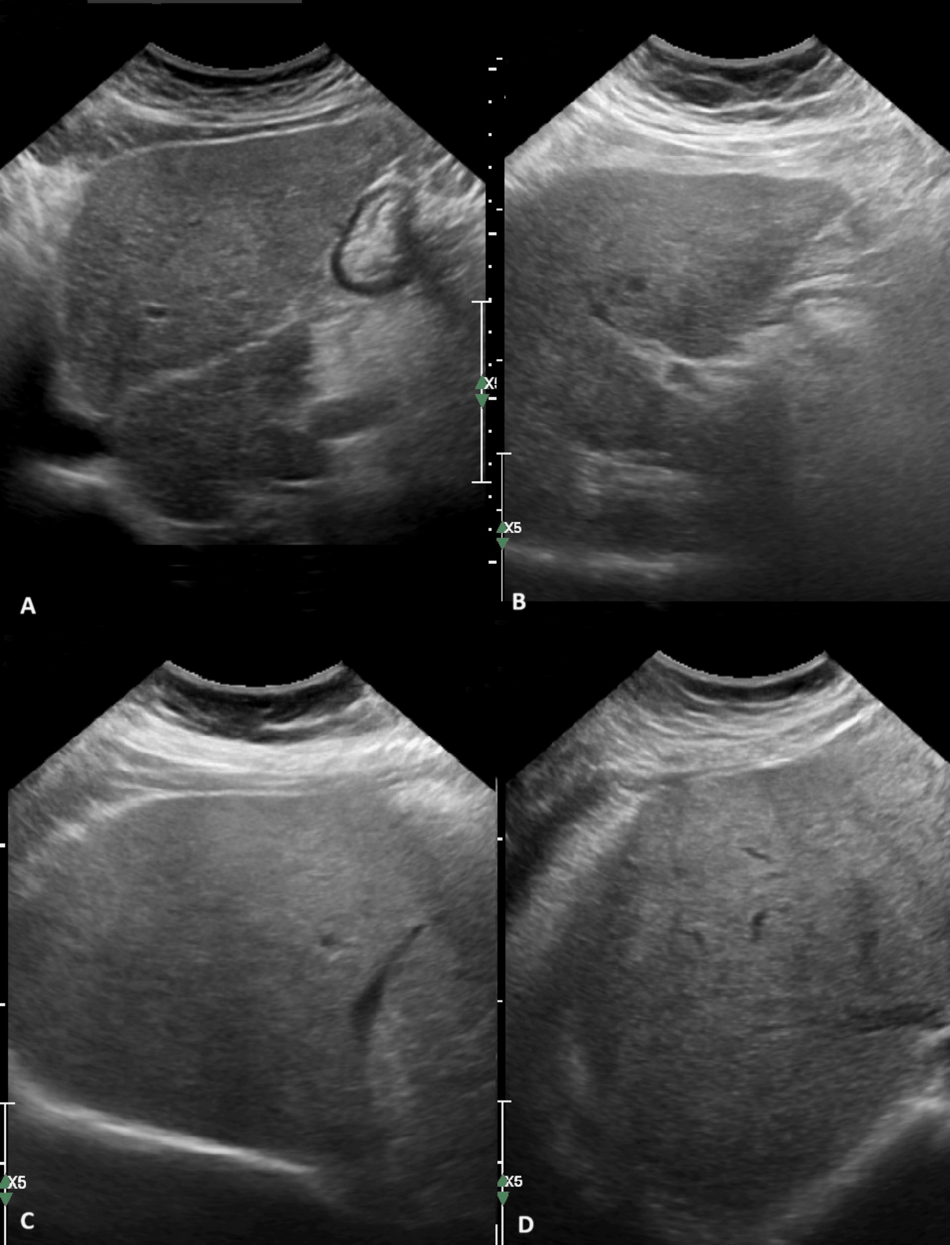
Ultrasound of the abdomen revealed hypertrophy of the left lobe (A, B) and hepatomegaly (C, D) with surface nodularity. Ascites was not observed.
